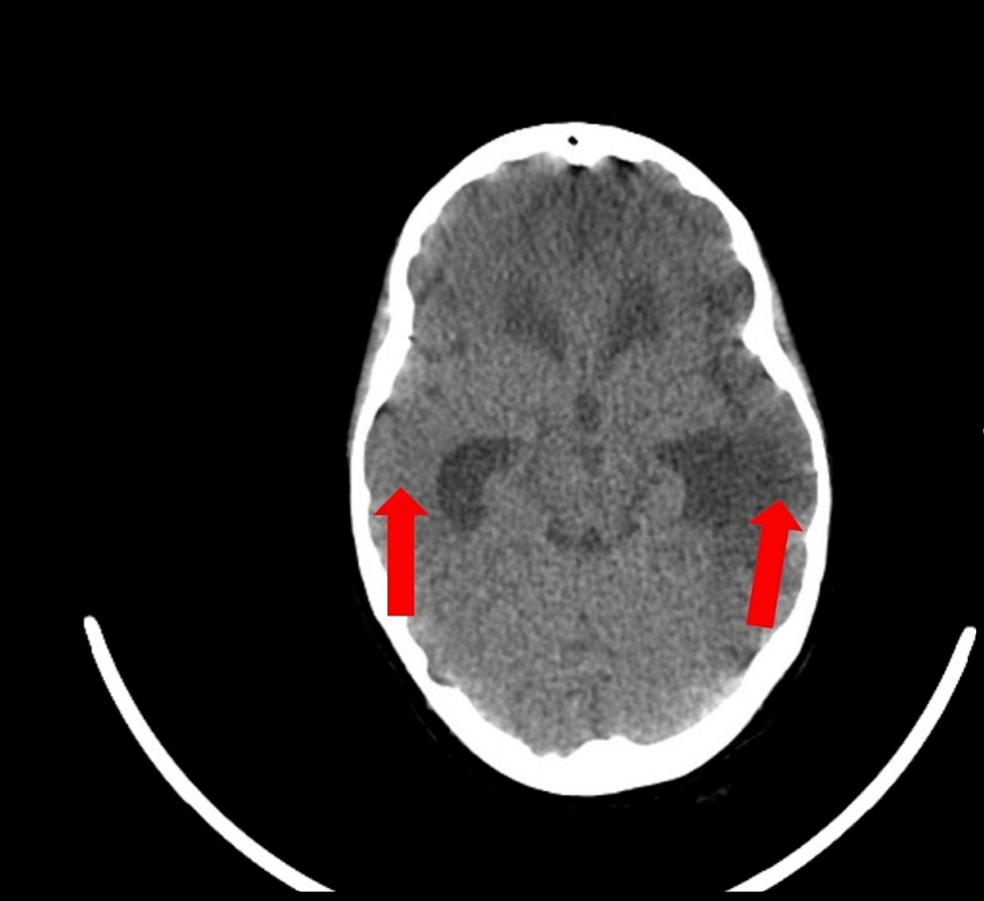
Cerebral CT scan revealed ischemic lesions in the right temporal and left hemispheric cortico-subcortical topography.
